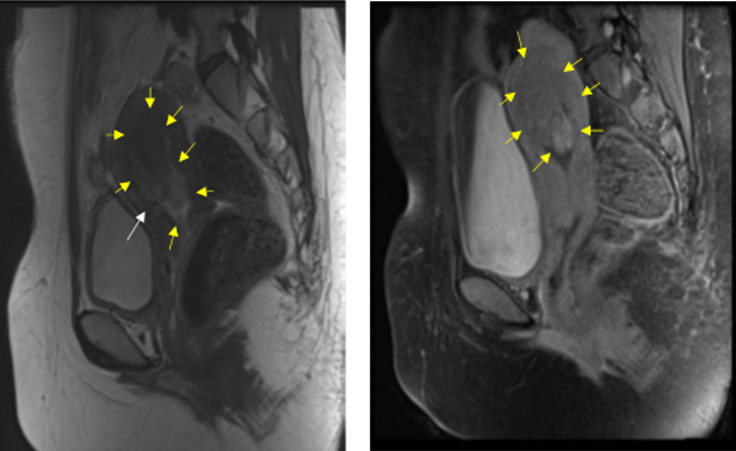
MRI after radiotherapy; the yellow arrows indicate the tumor which has reduced in size, no longer protruding through the cervix
